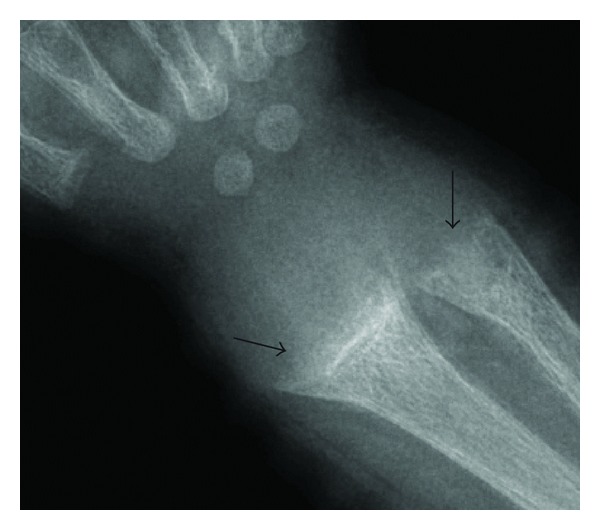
Wrist radiograph of the same patient as Figure 1. The distal metaphysis of the radius and ulna is widened and frayed with a mild cupped configuration (arrows).
